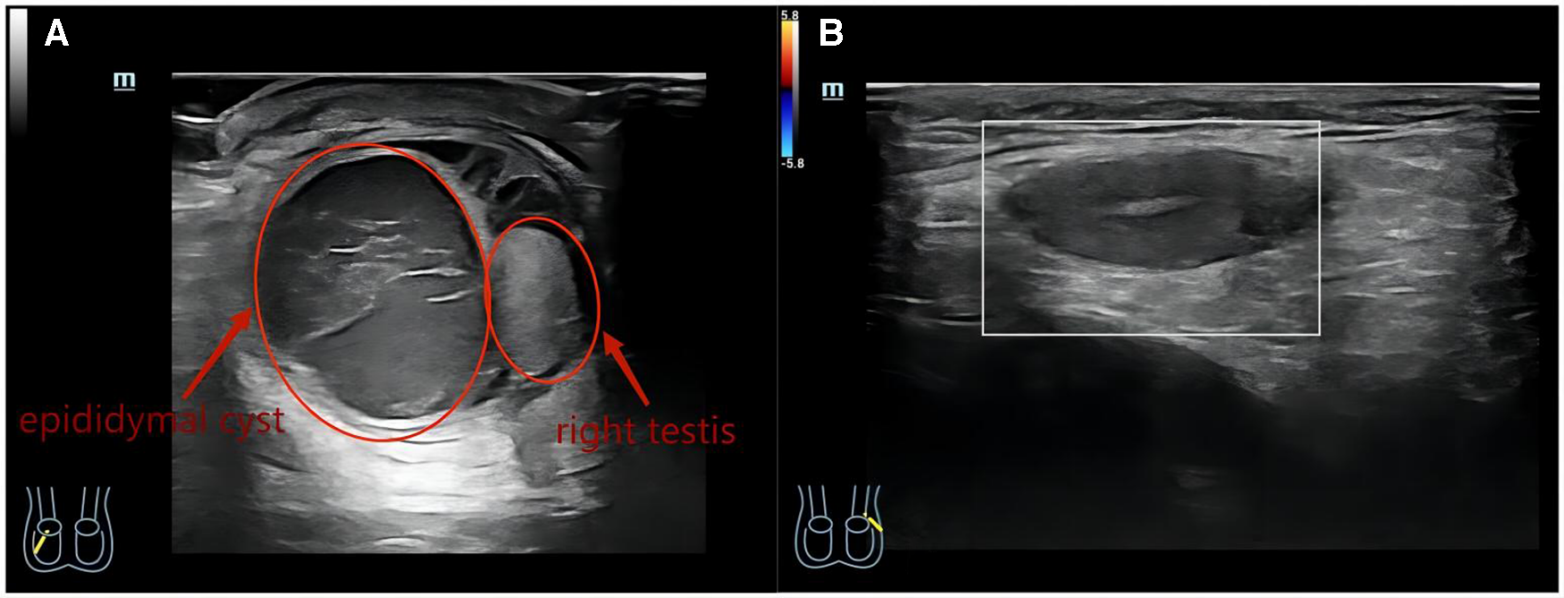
(A) Ultrasound image of right testis with epididymal cyst (on acute presentation). (B) Ultrasound image of the left testis in the left inguinal area.
